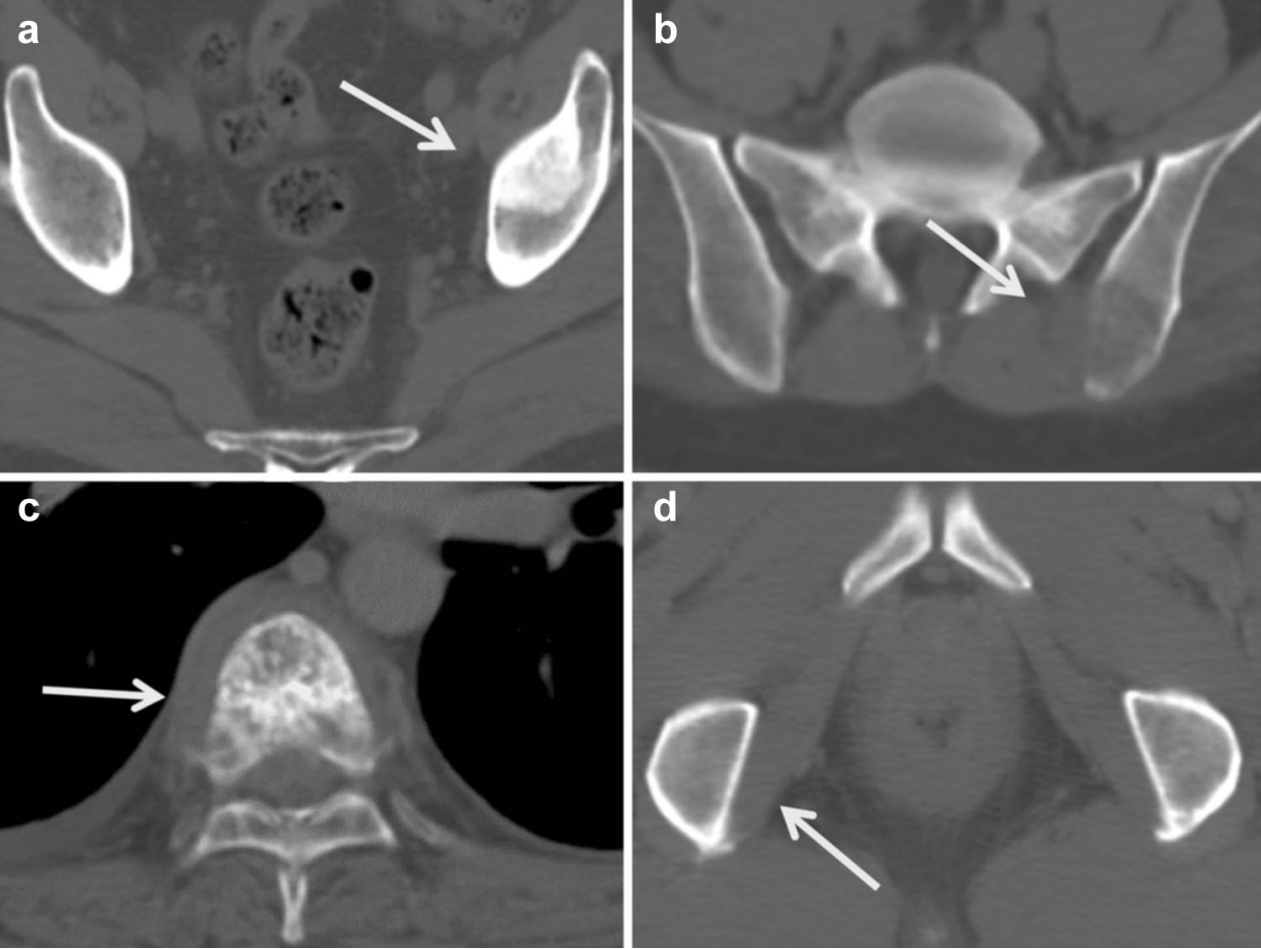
Representative examples of the various computed tomography (CT) morphologies: a osteoblastic pattern (left ilium); b osteolytic pattern (left ilium at the iliosacral site); c mixed osteolysis and osteoblastic pattern (lumbar vertebra); and d invisible pattern (right ischium). On a later CT scan of the invisible patient, the osteoblastic lesion has been developed. *Arrows* indicate osseous metastatic sites
